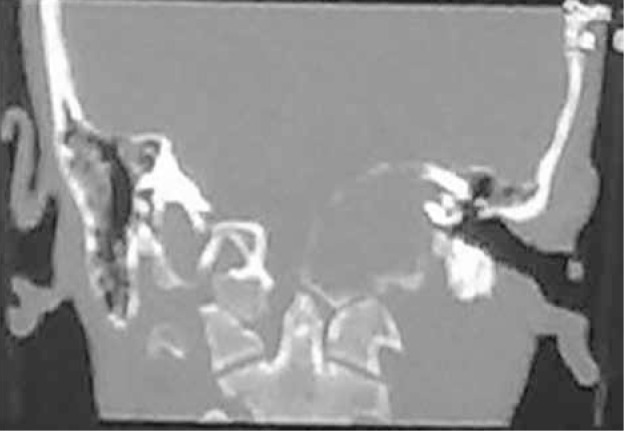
Coronal skull base CT scan Lytic expansile mass on the left side of the hypoglossal foramen extended to jugular foramen and internal auditory canal.
